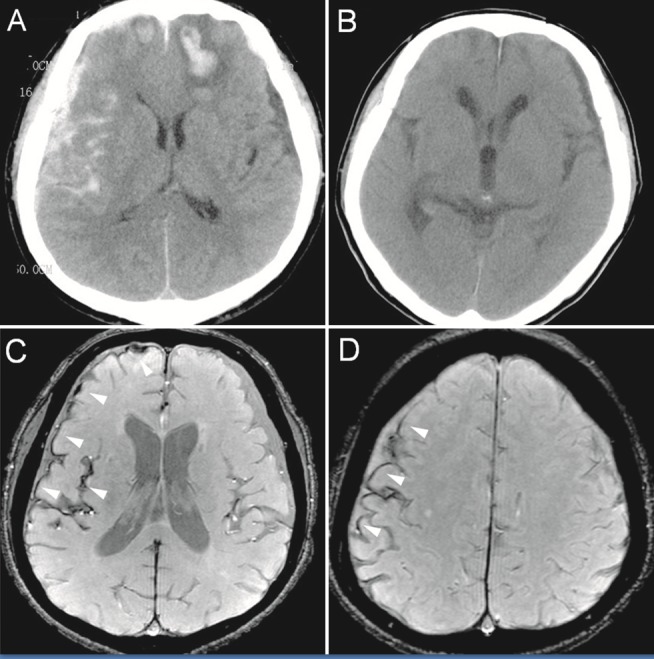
CT and enhanced gradient echo T2 star-weighted angiography (ESWAN) images of the brain of a 54-year-old man who experienced a traumatic brain injury. An axial head CT image displays right frontotemporal SAH (Fisher grade 4) with bilateral frontal contusions and intracerebral hematoma (A). A follow-up CT image 26 weeks after the brain injury indicates that the hemorrhages were completely resolved and the lateral ventricles were mildly enlarged (B). A follow-up MRI (1.5T) image was obtained 26 weeks following the head injury (C,D). The axial ESWAN image displays a rim of hypointensity (*arrowheads*), with hemosiderin deposits forming along the cerebral convexity (C, D).
